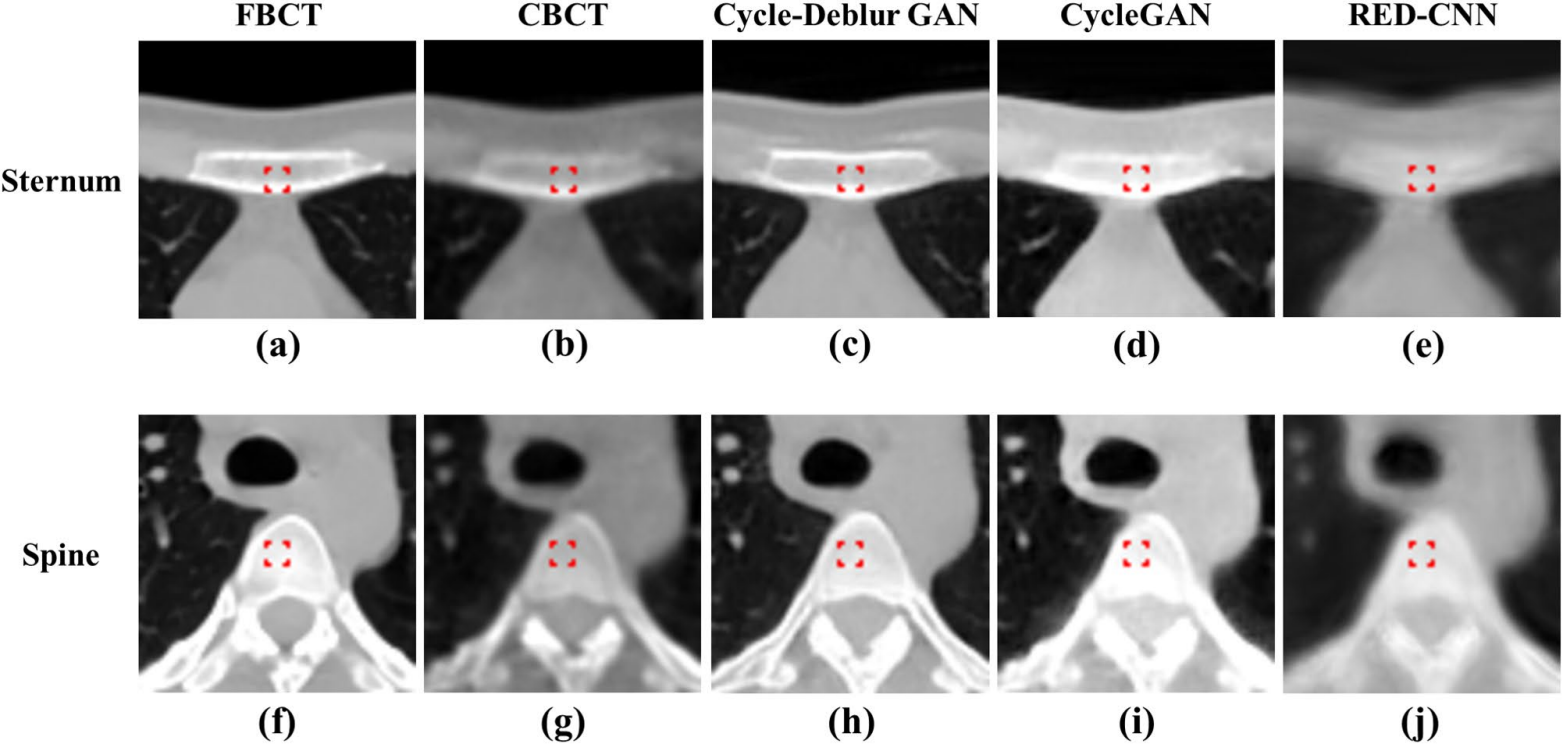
The bony structure ROIs of the sternum and spinal process for quantitative evaluation are shown in (a,f): FBCT, (b,g): CBCT, (c,h): Cycle-Deblur GAN, (d,i): CycleGAN and (e,j): RED-CNN were performed by XVI software and PMOD software. (W = 1450, L = − 225 for all CT images).
